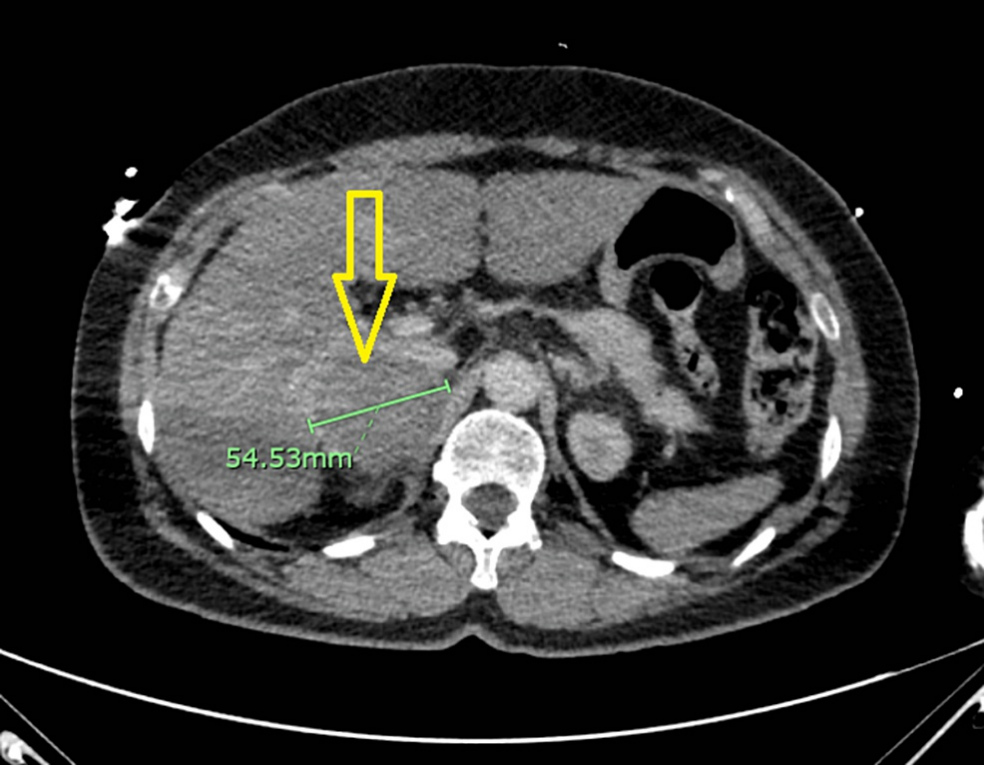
CT scan abdomen: showed 5.4 cm mass (yellow arrow) in the right adrenal gland highly suspicious for pheochromocytoma CT: computed tomography
